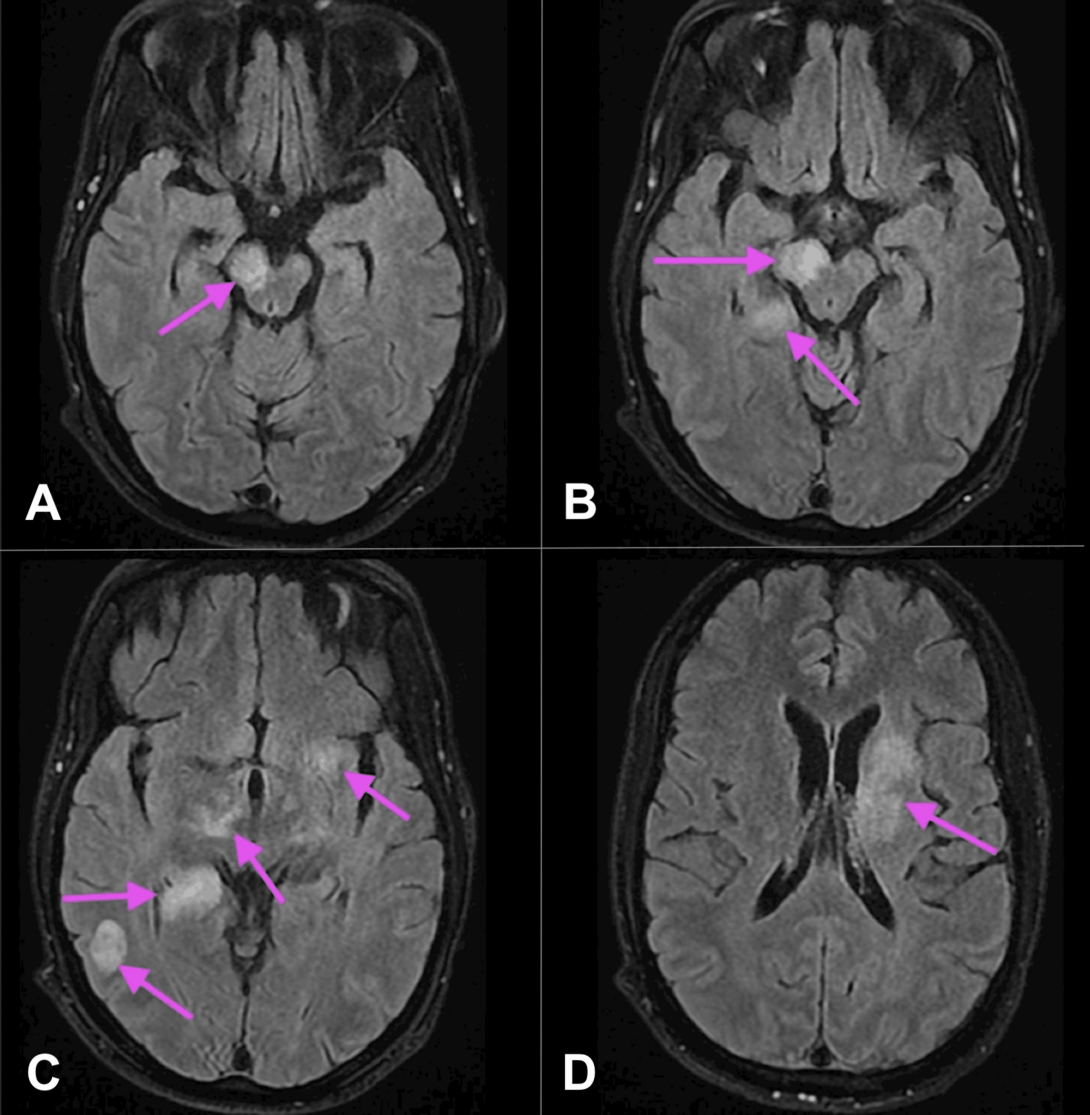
T2 FLAIR MRI brain with and without contrast (A-D) Differing axial view depths of the brain. A repeat MRI of the brain using T2 FLAIR with and without contrast shows active demyelination in the left dorsal medulla, the posterior right hippocampus, the right temporo-occipital subcortical white matter, and both the left corona radiata and basal ganglia. Large chronic demyelinating lesions are noted within the right midbrain, cerebral peduncle, and caudal thalamus. Small chronic demyelinating lesions are seen within the left thalamus and internal capsule. Arrows highlight the multiple areas of demyelination. FLAIR: fluid-attenuated inversion recovery; MRI: magnetic resonance imaging
